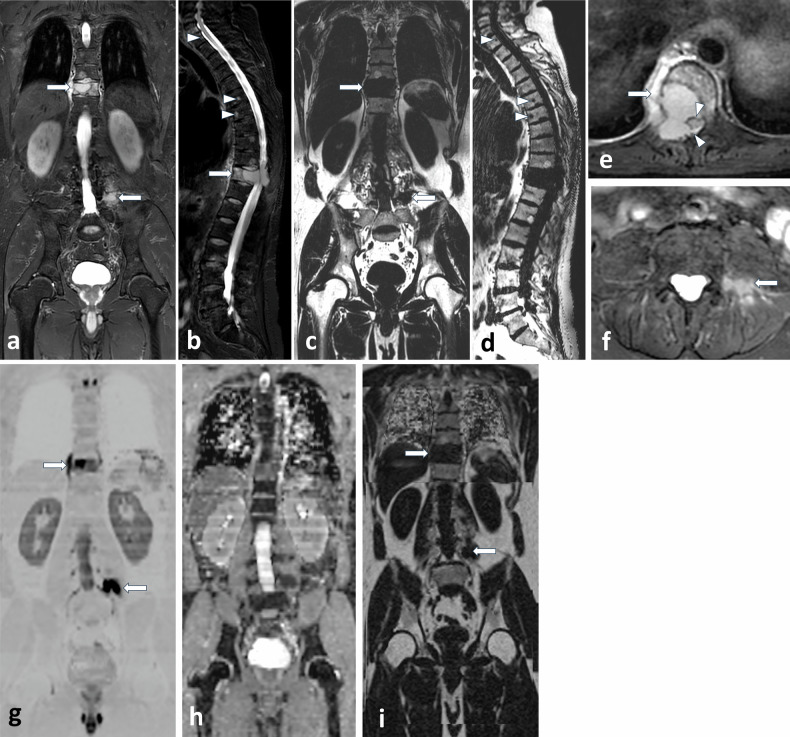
Whole body MRI (WB-MRI) protocol for bone marrow imaging in a 67-year-old patient with newly diagnosed multiple myeloma. a–d 3D Fast spin echo (FSE) T2 Dixon sequence: water-only (a, b) and fat-only (c, d) reformatted coronal and sagittal images obtained from the same isotropic acquisition show two tumoral foci within the T10 vertebral body and left transverse process of L4 (arrows). Smaller foci of low signal intensity on T1 and high signal intensity on T2 images are seen on the sagittal images (arrowheads in b and d). This sequence also provides in-phase images for visceral study. e, f Transverse reformatted water-only images show the T10 and L4 lesions (arrows), and most importantly, the canal extension of the T10 focus (arrowheads in e). g, h DWI sequence: reformatted coronal high b-value image (inverted grayscale window, b = 1000 s/mm^2^) shows the T10 and L4 lesions (arrows in e). Apparent diffusion coefficient (ADC) map (f) is calculated for lesion ADC follow-up after treatment. i Fat fraction map (coronal reformat) is also acquired for treatment response assessment; note the visibility of the tumoral foci (arrows)
